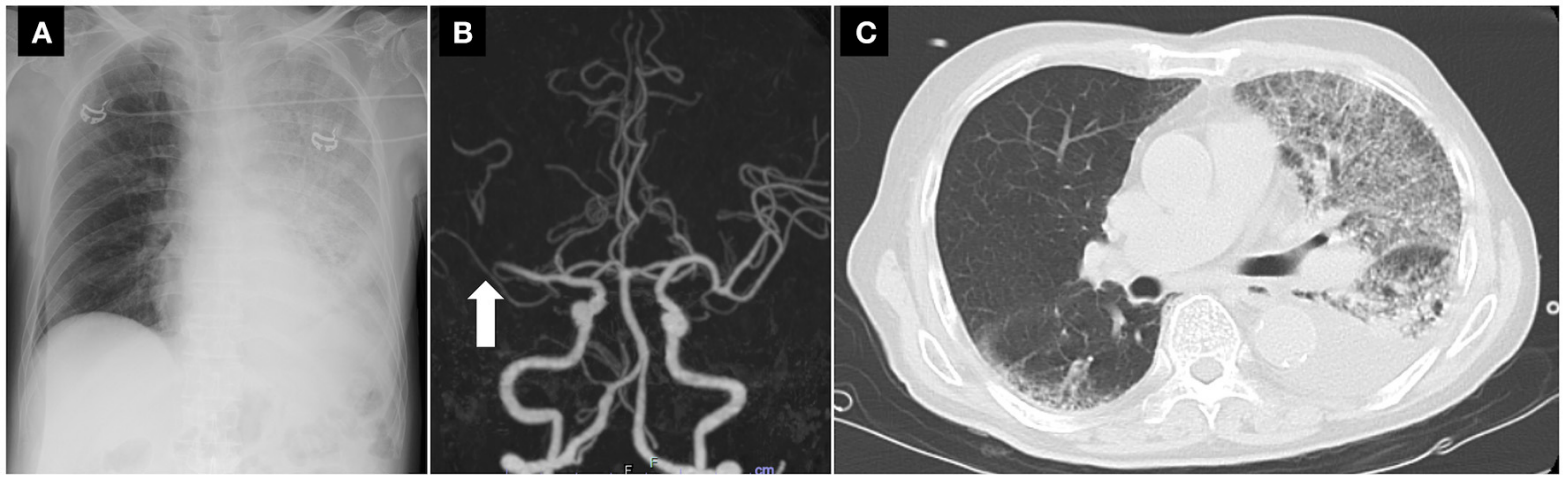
A portable X-ray, CT angiography, and chest CT findings of the stroke patient with suspected COVID-19. (A) A portable chest X-ray showing extensive consolidation in the left lung. (B) CT angiography showing an occlusion of the distal portion of the right middle cerebral artery (*arrow*). (C) Chest CT showing extensive consolidation in the left lung and pleural effusion in the left side. CT, computed tomography.
